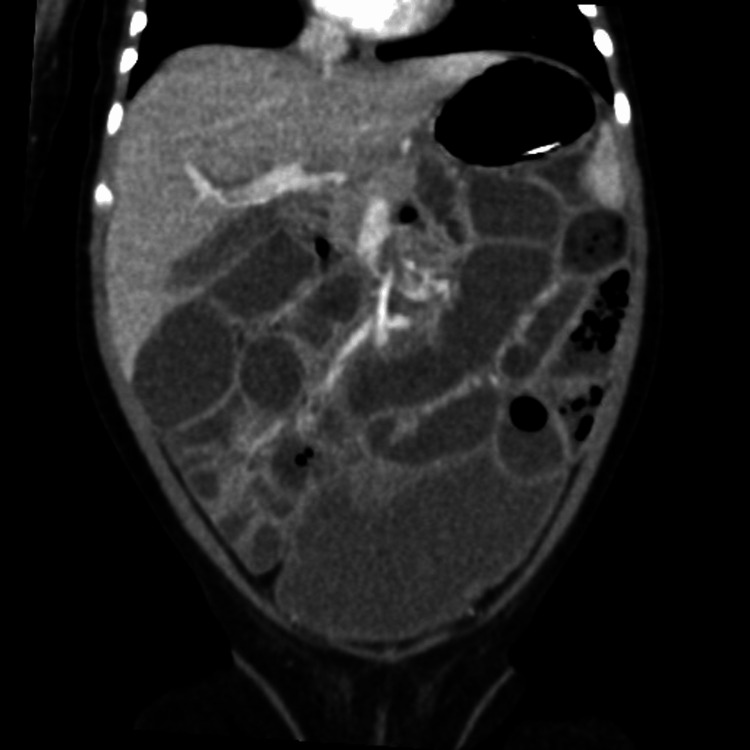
CT of the abdomen (coronal view).
